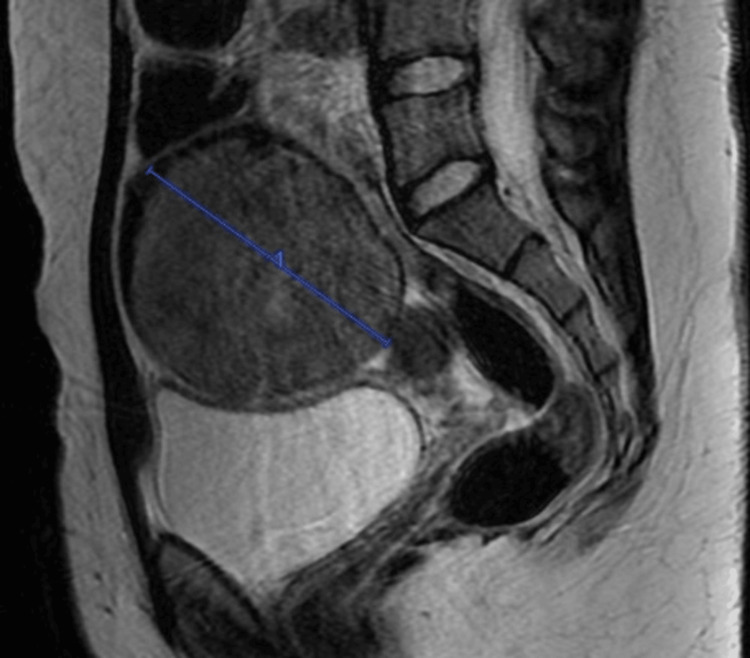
MRI (sagittal view) showing a 7.6 x 8.7 x 11.6 cm solid mass with tiny cystic components in the region of the right adnexa that crosses the midline into the left adnexal region
